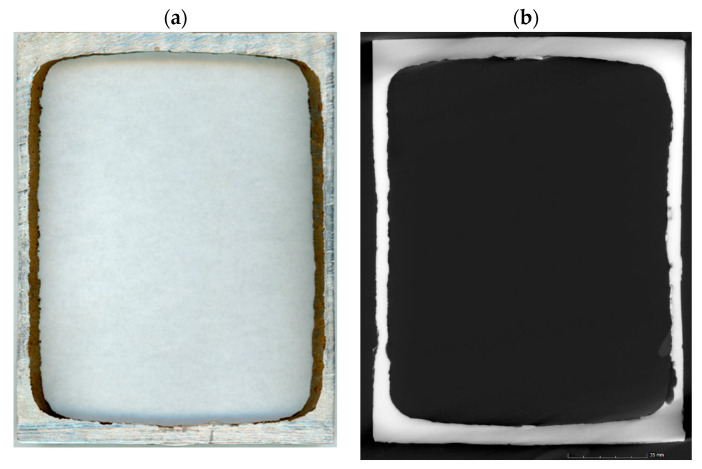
Guide cross section (a) and CT scan (b).
